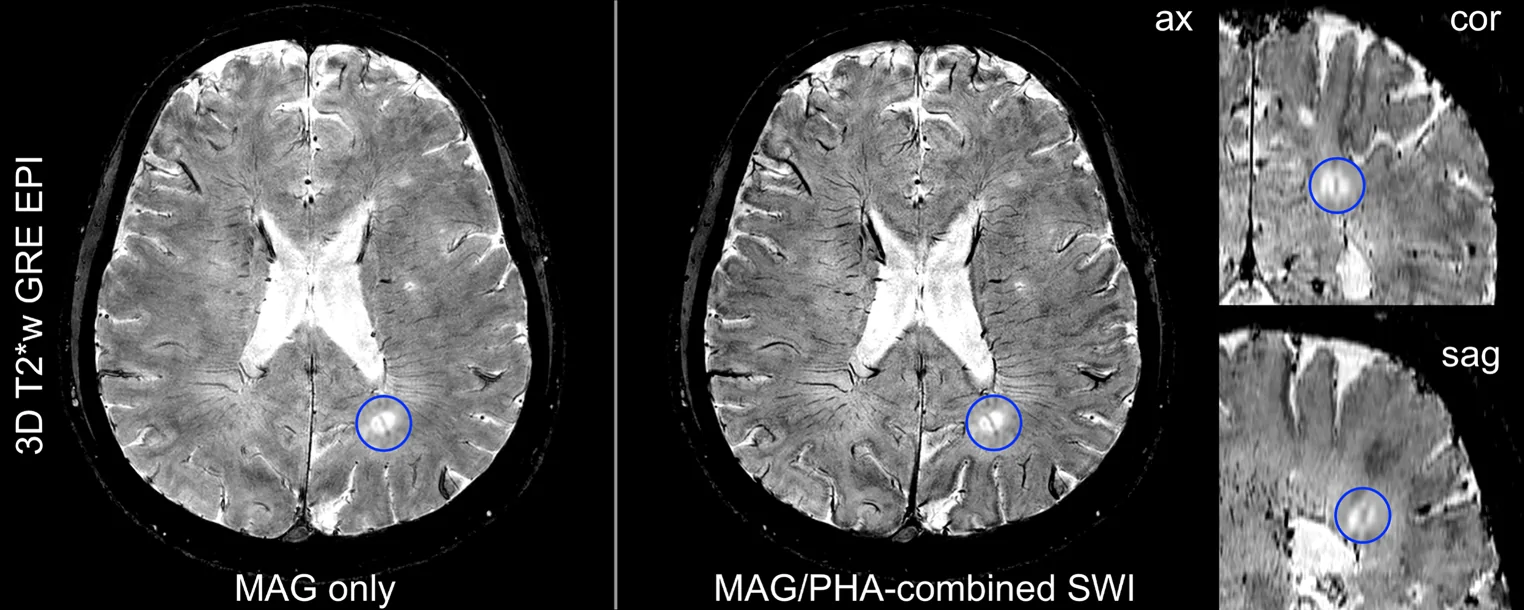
Detection of the central vein sign (*CVS*) as a new diagnostic MRI biomarker for MS by susceptibility-weighted imaging (*SWI*). CVS (blue circled), meeting the required identification/exclusion criteria (Table 4) and confirmed in three perpendicular planes by high-resolution 3D T2*w GRE EPI (0.44 × 0.44 × 1.00 mm^3^) in a patient with proven MS. Note that SWI improves CVS and, in general, transmedullary vein conspicuity compared to magnitude (*MAG*) only reconstructions because processed phase (*PHA*) is venographic. Therefore, CVS should be evaluated on MAG/PHA-combined SWI (Table 6). Note that 3D GRE EPI can also output processed PHA images for paramagnetic rim lesion (*PRL*) detection (cf. Fig. 3). We recommend using the same SWI for CVS and PRLs, resorting to the sequence most easily available on site and optimized for that purpose (cf. Table 3). Data courtesy of Thomas Illigen, Siemens Healthineers, images generated by AJB. Future product availability cannot be guaranteed.
